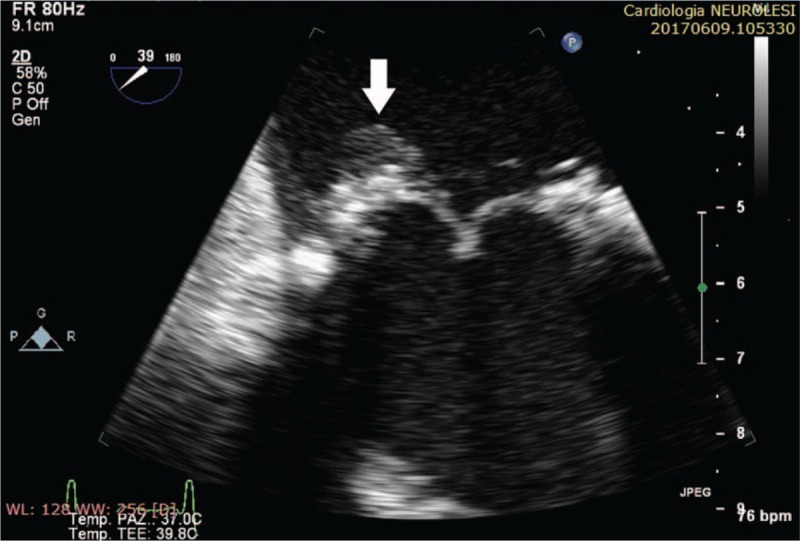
Transesophageal echocardiography shows a great vegetation on posteromedial portion of the mitral prosthetic ring. LA = left atrium.
